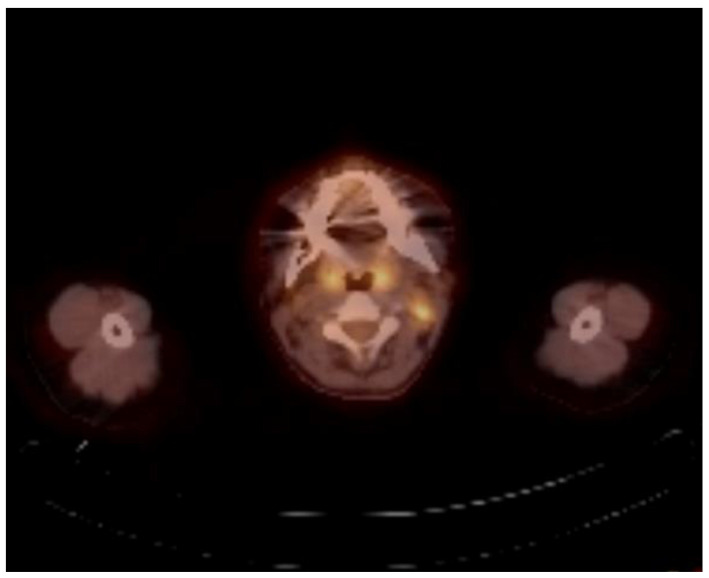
F-18FDG PET/CT imaging shows increased metabolic activity and FDG uptake in left level 2 cervical lymph nodes.
